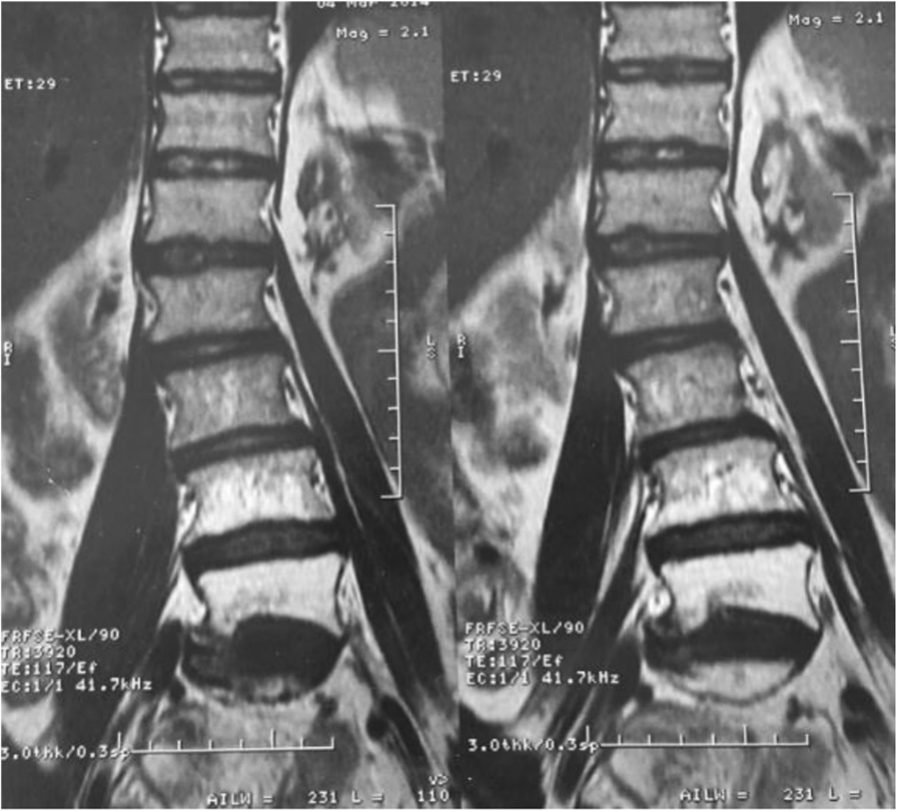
Spinal MRI T2-weighted sequence
